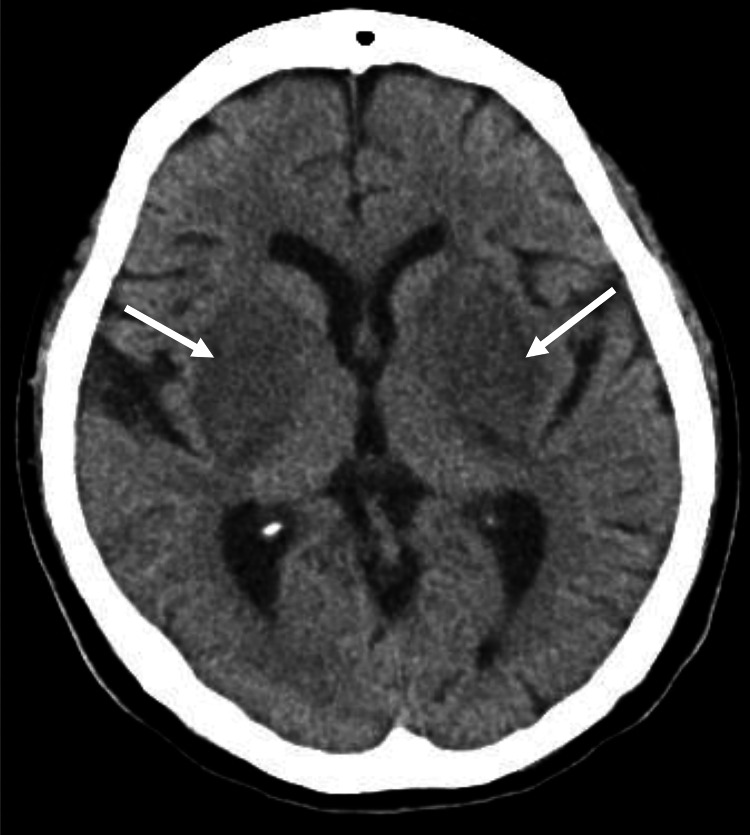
Brain computed tomography performed in the emergency department Low density area were observed in the bilateral basal ganglia (arrows)
